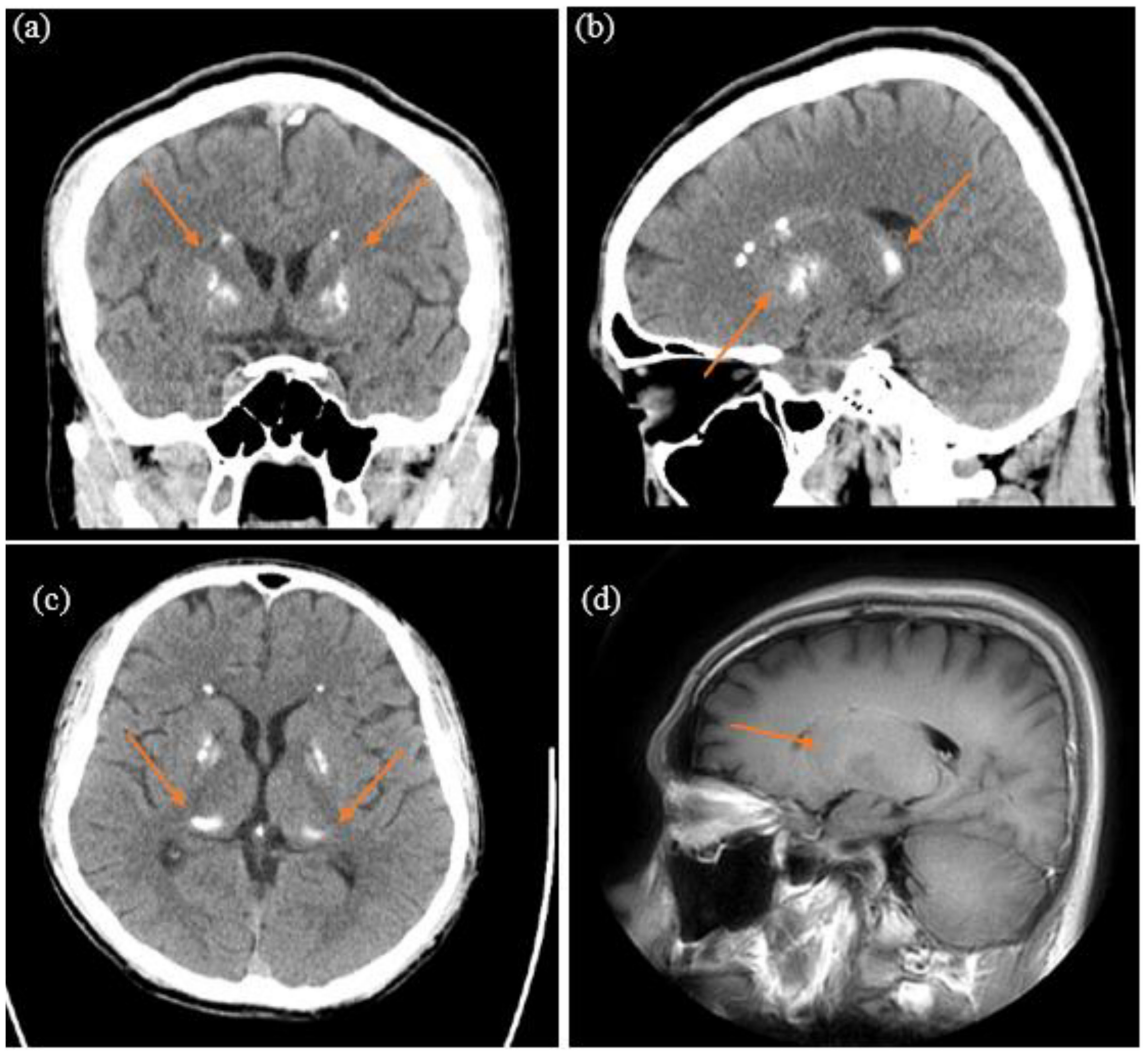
Basal ganglia calcification in the brain computed tomography: (A) coronal plane; (B) sagittal plane; (C) transverse plane. Basal ganglia calcification in the brain magnetic resonance imaging: (D) sagittal plane.
